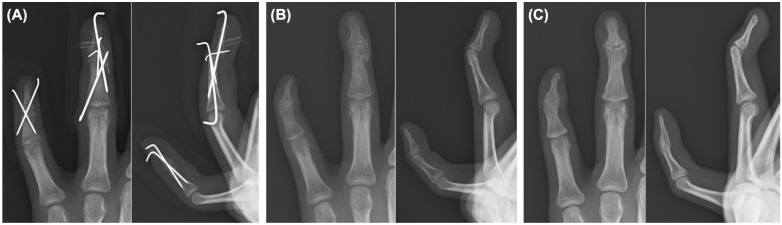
Serial radiographs of the left ring and little fingers. (A) Radiographs obtained at 6 weeks, before hardware removal, showing maintained alignment. (B) Radiograph obtained at 3 months, showing progression of fracture healing. (C) Final radiograph obtained at approximately 21 months, showing union and final alignment of the ring and little fingers.
